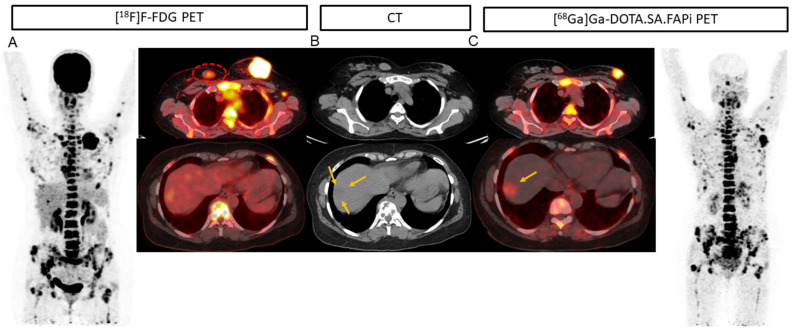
[^18^F]F-FDG PET/CT (A) and [^68^Ga]Ga-DOTA.SA.FAPi PET/CT (B) avid soft tissue density mass measuring 5.2 × 4.3 cm noted in the upper quadrant extending into the retro-areolar region and upper quadrants involving nipple-areola complex and overlying skin. A small well defined rounded soft tissue density lesion measuring 2 × 3 cm was noted in the upper inner quadrant of the right breast with mild FDG uptake-likely fibroadenoma (A, red dotted circle) but no uptake on [^68^Ga]Ga-DOTA.SA.FAPi scan. Due to increased physiological hepatic FDG uptake, the liver lesion (A) is masked in the^18^F-FDG PET/CT as compared to the [^68^Ga]Ga-DOTA.SA.FAPi PET/CT [(C), yellow arrow] and corresponding lesion on CT [(B), yellow arrows].
